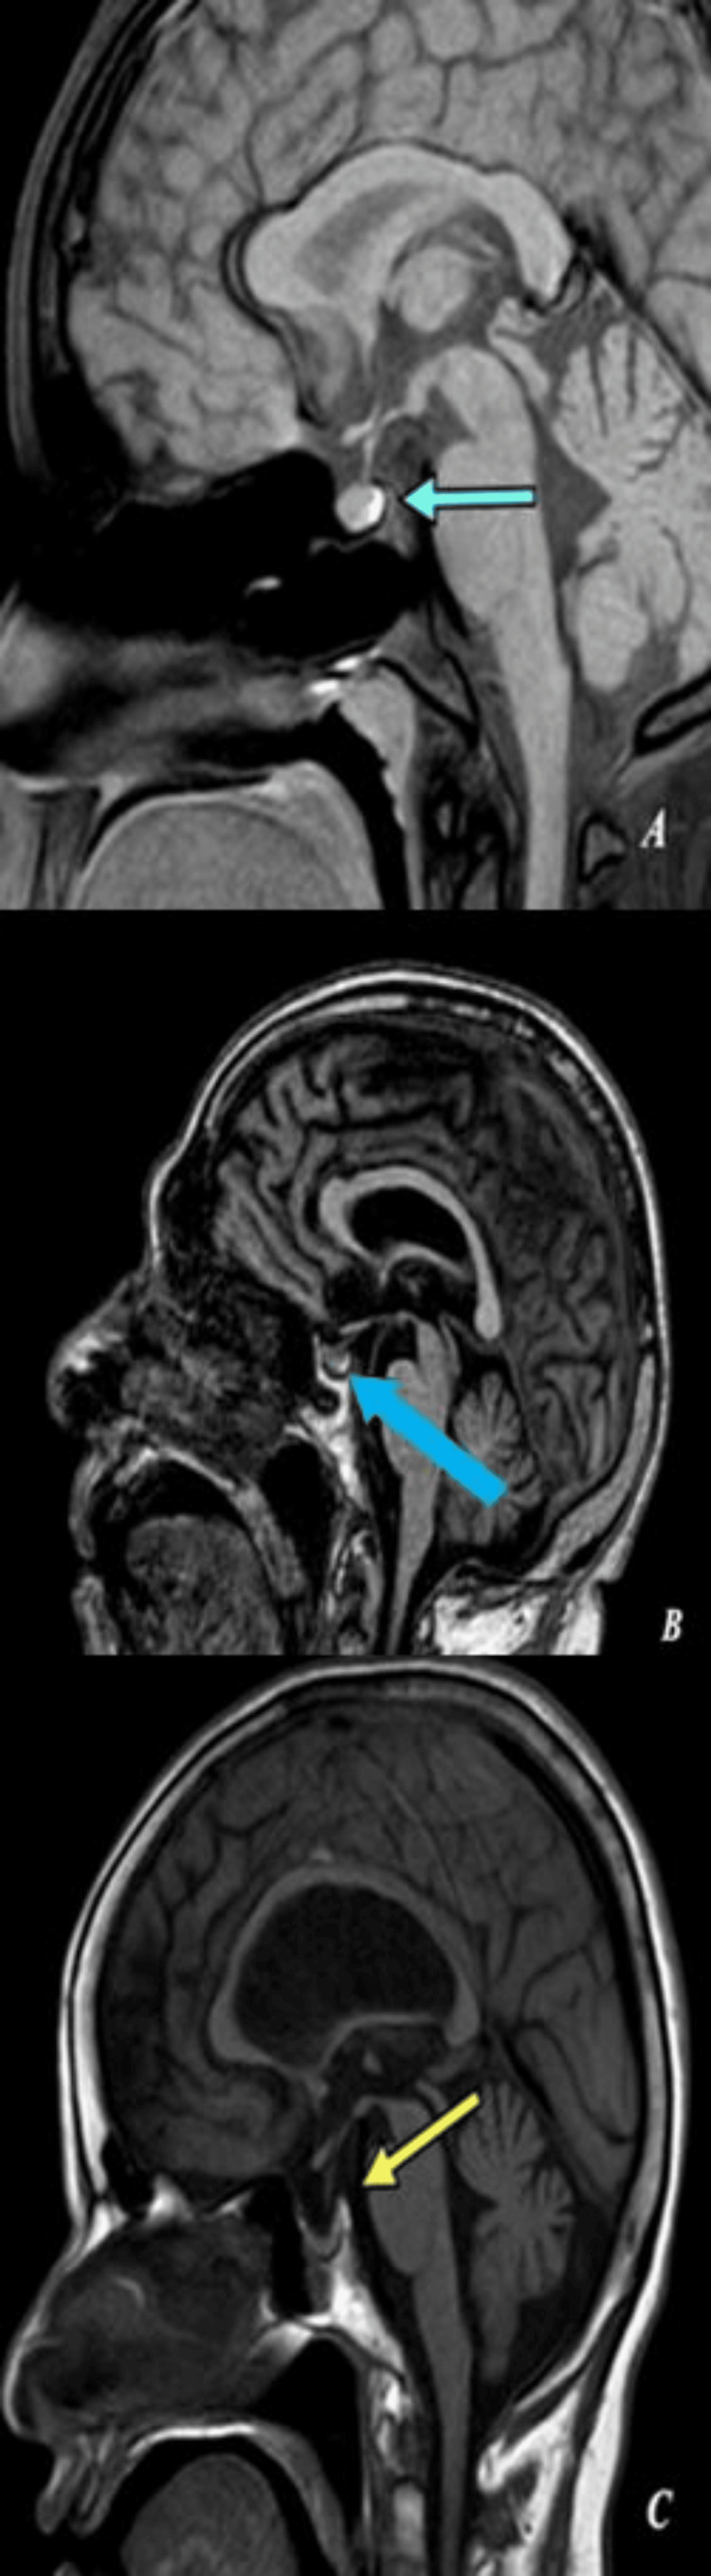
(A, B, C): MRI of the sella turcica. (A) T1 mid-sagittal: the pituitary gland in a 20-year-old male shows that the upper border is convex in shape (green arrow). (B) T1 mid-sagittal: the pituitary gland in a 62-year-old male shows that the upper border is flat in shape (blue arrow). (C) T1 mid-sagittal: the pituitary gland in a 55-year-old female shows that the upper border is concave in shape (yellow arrow)
